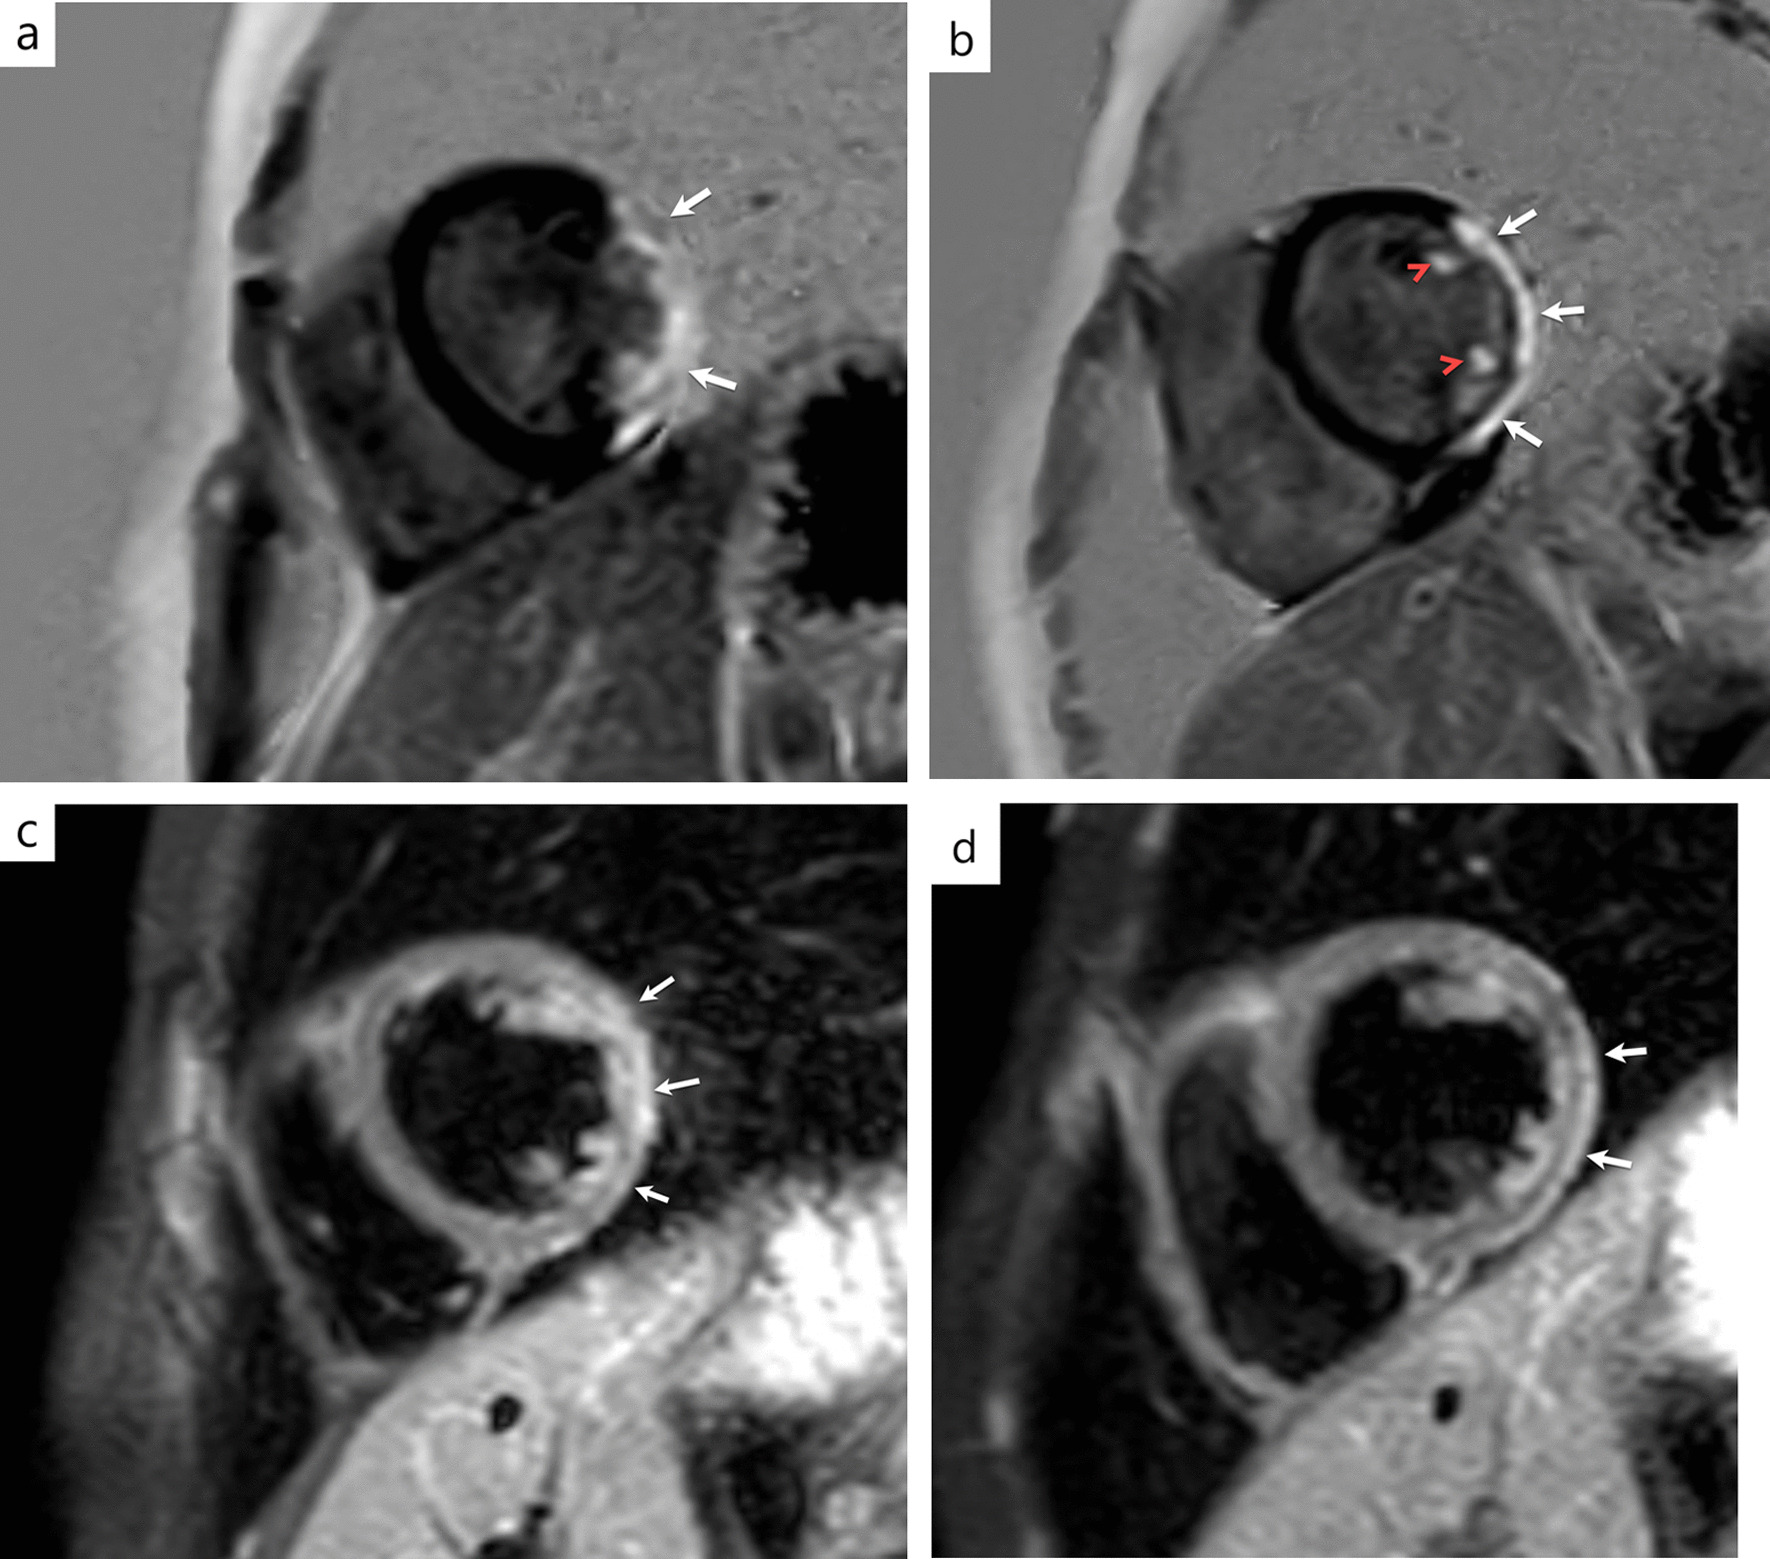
a–d Short-axis late gadolinium-enhanced cardiac magnetic resonance (MR) images with phase-sensitive inversion recovery (PSIR) demonstrate mid-wall to subepicardial late gadolinium enhancement at the basal to the mid inferior lateral wall (a and b, arrows) with papillary muscle enhancement (b, arrowheads), corresponding hyperintensity on T2-weighted imaging (c and d, arrows)
